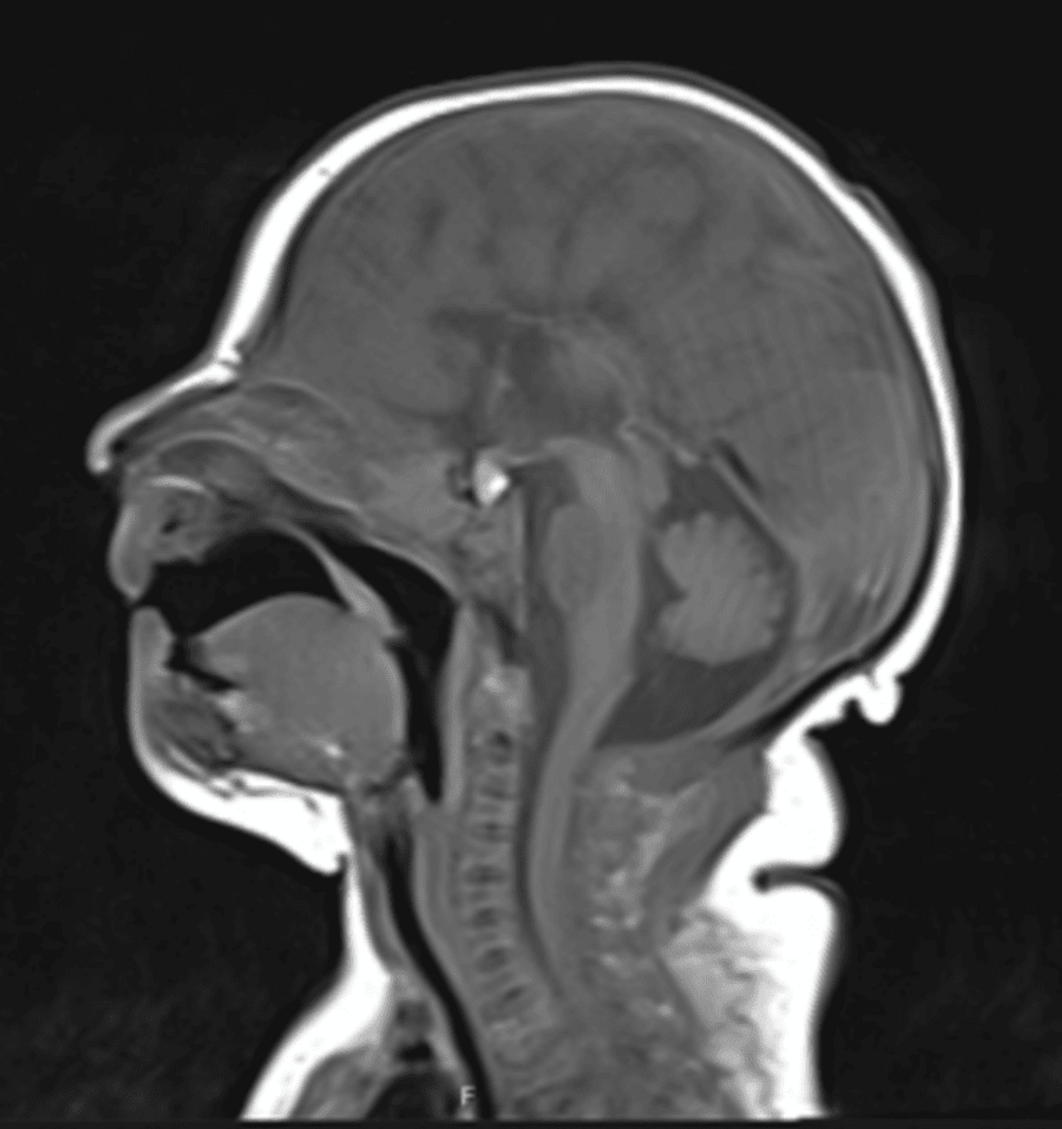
MRI brain (T1 sagittal) showing absence of the corpus callosum (motion artifact present).
